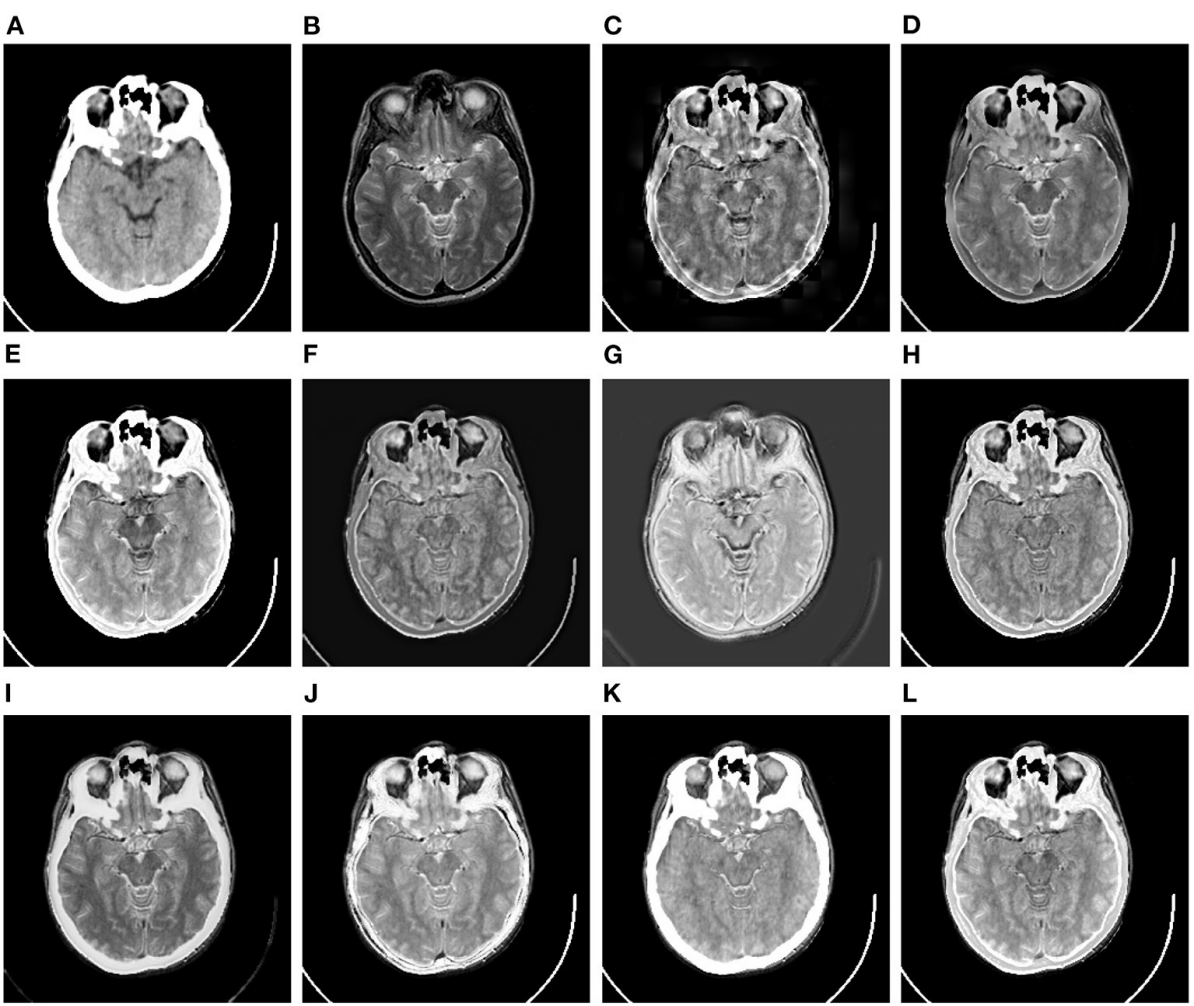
Comparison example on the CT-MR image dataset. (A,B) are the original CT image and MR image, respectively. (C–L) are the fusion results of DWT, GFF, LPSR, U2Fusion, DDcGAN, IFCNN, EMFusion, DRBIF, LEGFF_0_, and our method, respectively.
